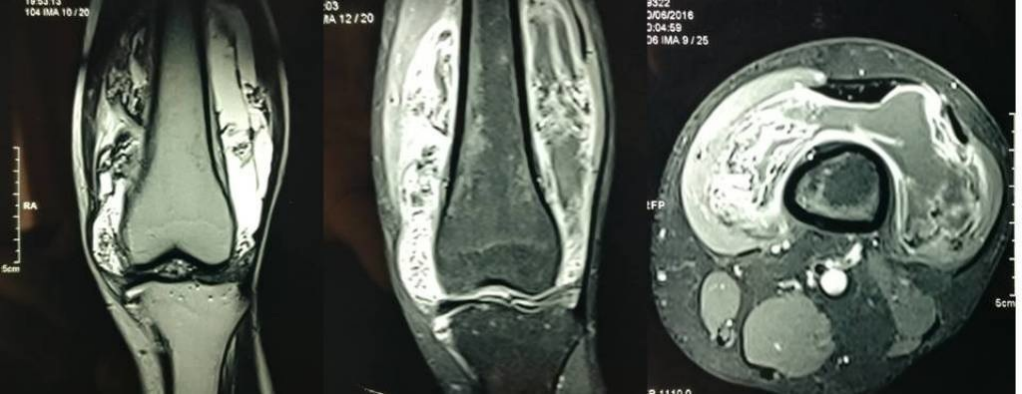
MRI of the knee joint - coronal and axial views Mulitlobulated lesions seen medially and laterally around the knee joint suggestive of PVNS.
